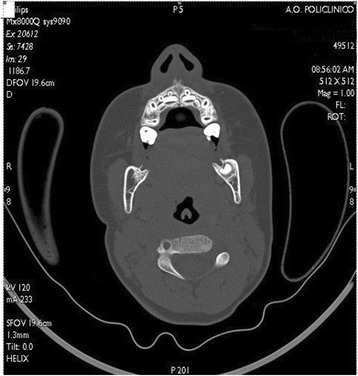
Computed tomography performed after chemotherapy, showing no sign of bone involvement with complete regression of the disease and a good position of the teeth involved in the neoplastic lesion
